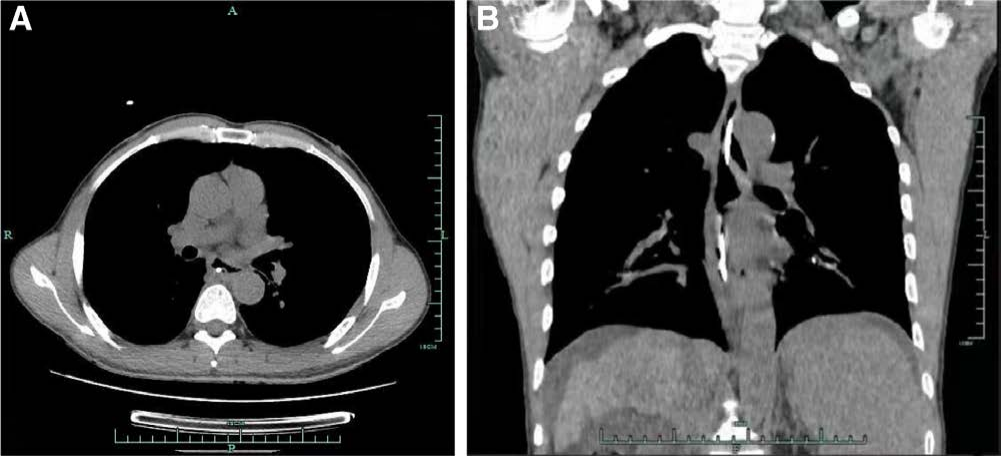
CT images. Axial view (A) and coronal view (B) indicating the disappearance of the esophageal mass and the improvement of esophageal stenosis. CT = computed tomography.
